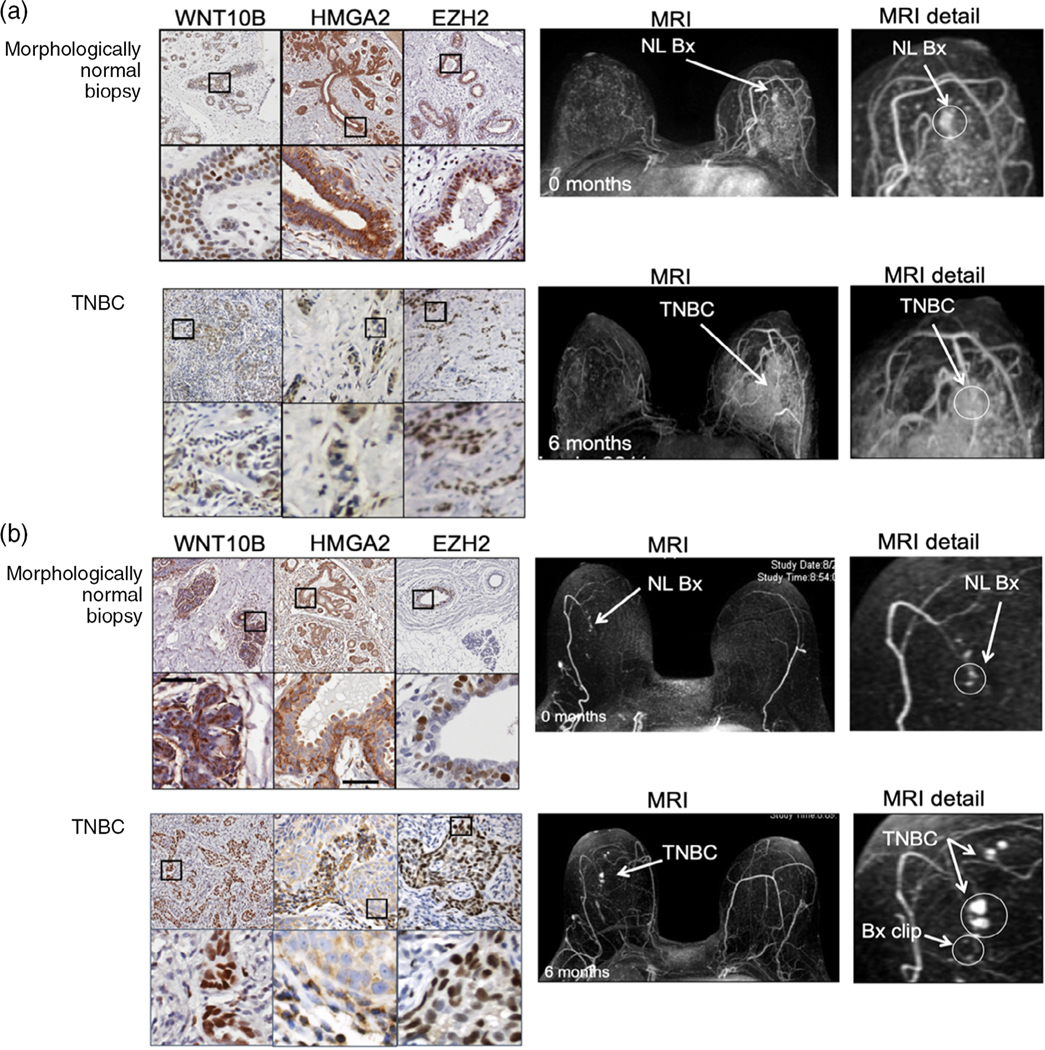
Matched MRI guided biopsy and MRI imaging demonstrates Wnt10B, HMGA2, and EZH2 expression in both morphologically normal biopsy at 0 months and follow up MRI imaging at 6 months. (a) 36-Year-old high-risk premenopausal woman and (b) 61-year-old high-risk woman had an abnormal screening MRI at 0 months and 6 months MRI follow-up. For both women, biopsy at 0 months demonstrated morphologically normal tissue that had high expression of Wnt10B/HMGA2/EZH2; 6 month follow-up biopsy demonstrated TNBC that also expressed high levels of Wnt10B/HMGA2/EZH2, both women had multiple lymph nodes that contained invasive TNBC but no metastatic disease. After greater than 5 years follow-up both women are alive and disease free
